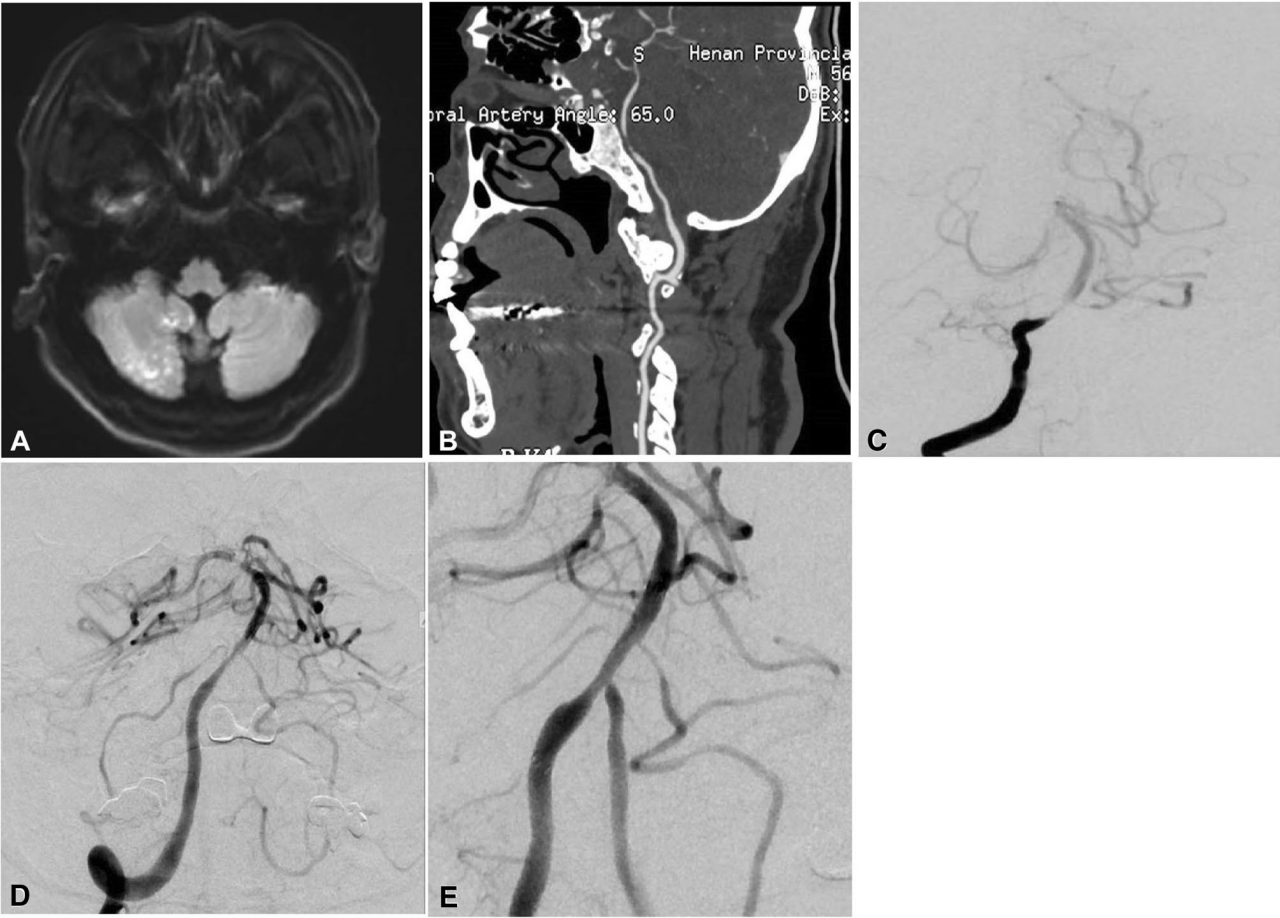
Severe stenosis at the lower segment of the basilar artery. (A) Magnetic resonance imaging (DWI) showed disperse lesions of new infarction in the right hemisphere of the cerebellum. (B) Computed tomography demonstrated severe stenosis at the lower segment of the basilar artery without calcification. (C) Digital subtraction angiography revealed severe stenosis at the lower segment of the basilar artery. (D) An Enterprise stent was deployed at the stenosis leading to patent flow. (E) Follow-up angiography at 6 months after stenting demonstrated good patency of the basilar artery at the lesion.
